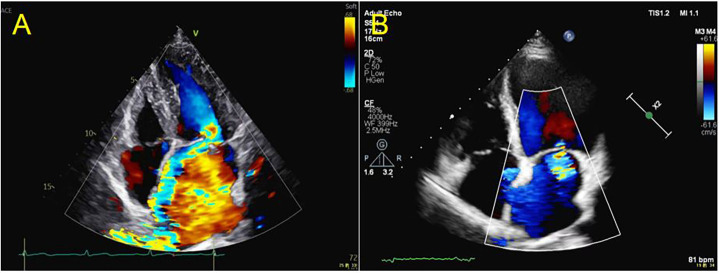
The echocardiography examination showing the AR and MR before
